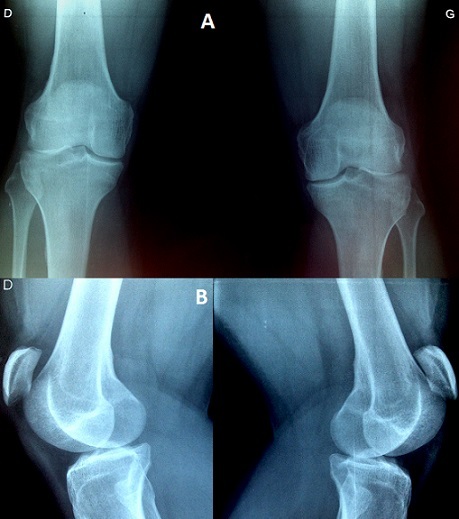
A) Bilateral frontal radiographs of the knee showing a patella alta; B) Bilateral lateral radiographs of the knee showing a patella alta
